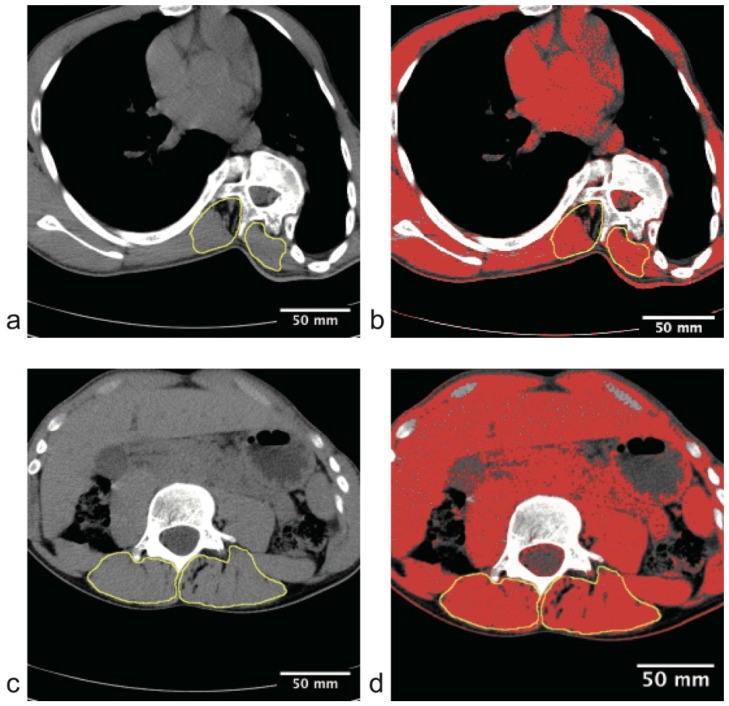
Representative CT images demonstrating segment-specific paraspinal muscle asymmetry in a patient with AIS. The paraspinal muscle areas are outlined by yellow circles. (a,b) Apical vertebral level. (b) Unenhanced axial CT image showing significant vertebral rotation. Note the visible hypodensity (darker areas) in the paraspinal muscles on the concave side (right), indicating greater low-attenuation signal. (b) Pseudo-color overlay where red pixels represent adipose tissue (−110 to −35 HU). The concave side exhibits extensive low-attenuation change compared to the convex side. (c,d) Stable vertebral level. (c) Axial CT image of the stable vertebra in the same patient, showing no rotation. (d) The pseudo-color map reveals symmetric muscle quality with minimal side-to-side difference (red pixels) on both sides, serving as an internal control. Note: This visually confirms that muscle asymmetry is localized to the deformity apex.
